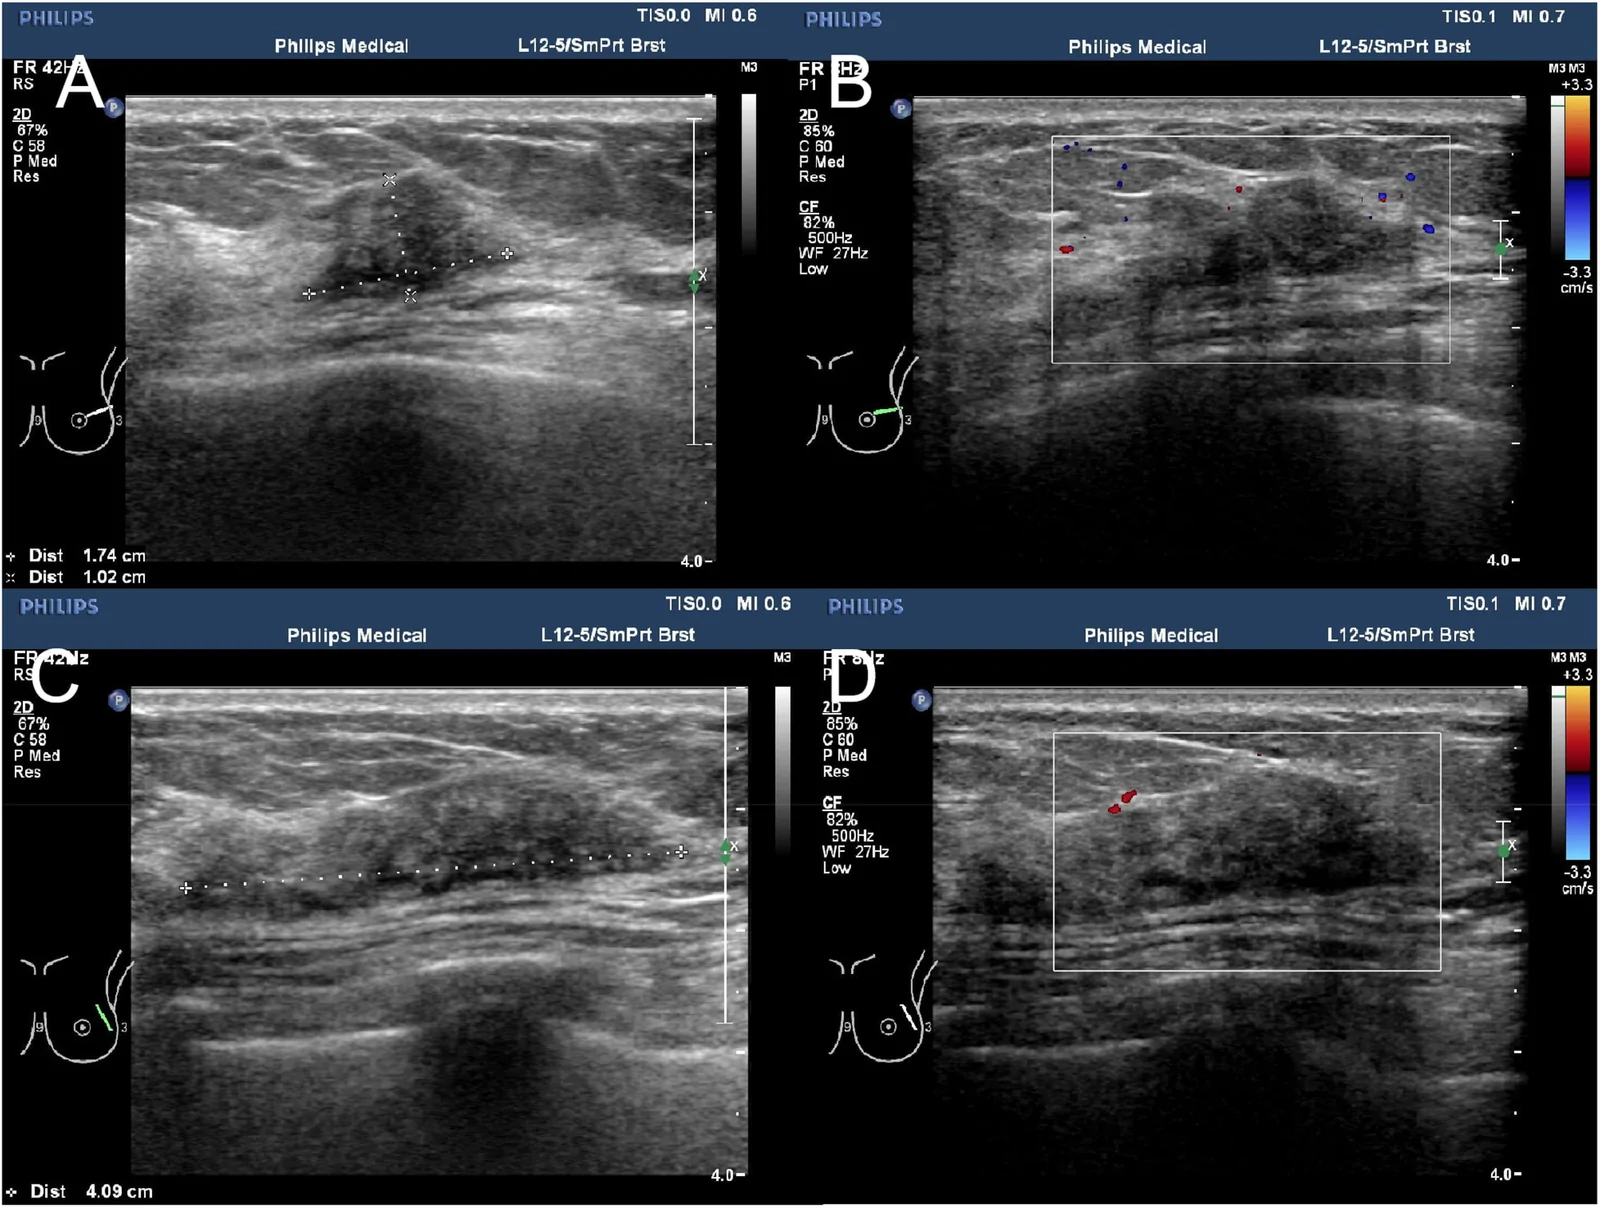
Left-breast ultrasonography in 2024. (A) An irregular heterogeneous hypoechoic focal component measuring approximately 1.74 × 1.02 cm. (B) Color Doppler imaging shows limited punctate flow. (C) A broader patchy hypoechoic abnormality extends approximately 4.09 cm. (D) Additional Doppler imaging demonstrates minimal vascularity within or around the abnormality.
